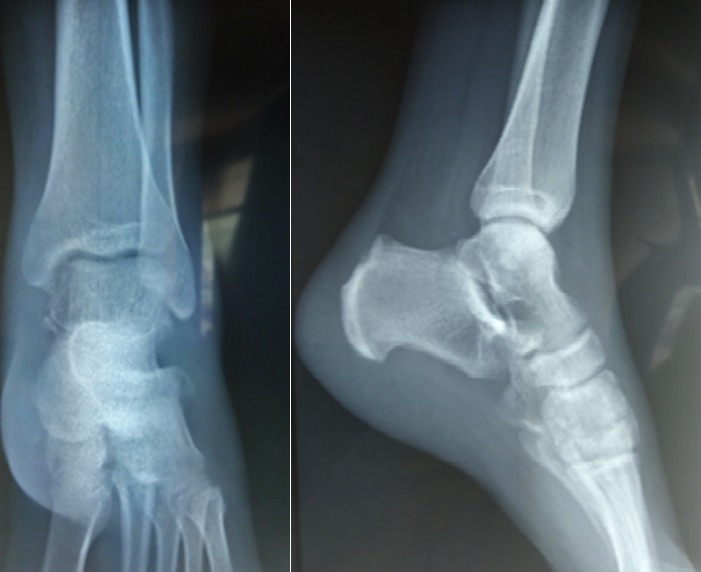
Radiographie après l’ablation du plâtre à 6 semaines
